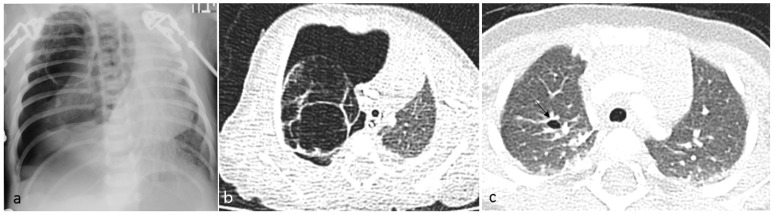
Six-day-old infant with respiratory distress. (a) Chest X-ray on day two of life: cystic lesion and tension pneumothorax on the right. (b) Chest CT without IV contrast performed on the same day, axial image: multicystic lesion and adjacent pneumothorax on the right hemithorax. (c) Chest CT performed 9 months later, with tiny cystic lesion on the right (arrow).
